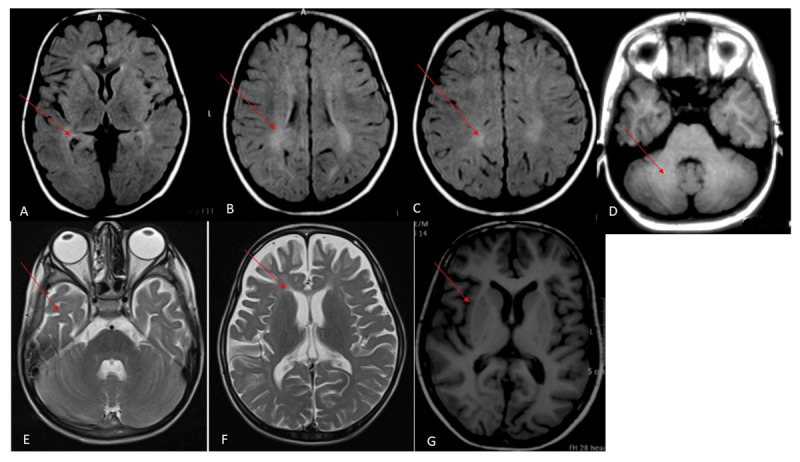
Brain MRI of 2 patients with late-infantile onset NPC (A-F); (A–C) Fluid-attenuated inversion recovery (FLAIR) axial images showing periventricular hyperintense signals (red arrow); (D) axial T1-weighted image showing normal cerebellum (red arrow); (E, F) Axial T2-weighted image showing periventricular hyperintense signals and fronto-temporal atrophy (red arrow); (G) Axial T1-weighted image of juvenile-onset NPC showing right lateral frontal atrophy (red arrow).
